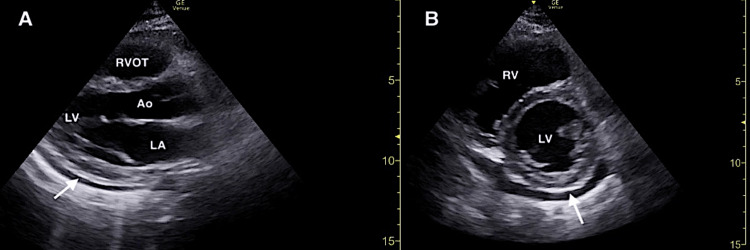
Focused cardiac ultrasound demonstrating a small pericardial effusion (solid white arrow) in the parasternal long (A) and parasternal short (B) windows. RVOT, right ventricular outflow tract; Ao, aortic root; LA, left atrium; LV, left ventricle
